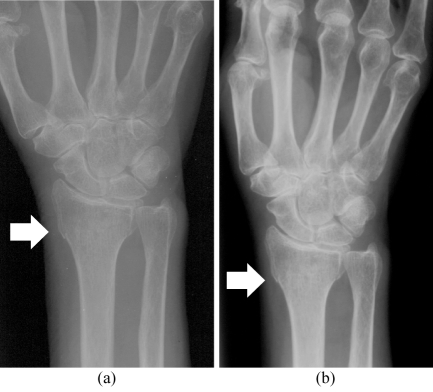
Image quality was better on the (a) MFC radiograph compared with (b) SFC radiograph. Soft tissue and outline of carpal bones are better depicted in MFC radiograph as well. Note that break in the cortex at distal radius with overlapping of fracture outline (arrow) is better appreciated in the MFC radiograph
